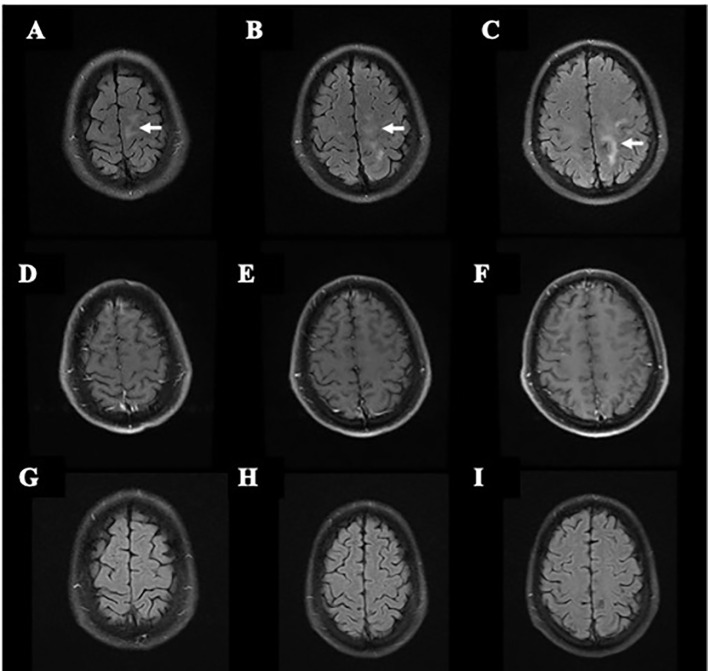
MRI findings in a 43-year-old man. (A–C) Axial T2-FLAIR images show patchy, irregular high-signal lesions in the left deep white matter and juxtacortical white matter (white arrow). (D–F) These lesions were not enhanced on axial T1-weighted images with contrast enhancement. (G–I) After chemotherapy and during the follow-up, axial T2-FLAIR images revealed that the previous high-signal lesions disappeared.
